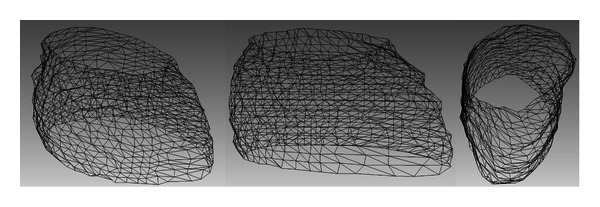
3D reconstruction of human heart from the segmented cardiac CT images.
